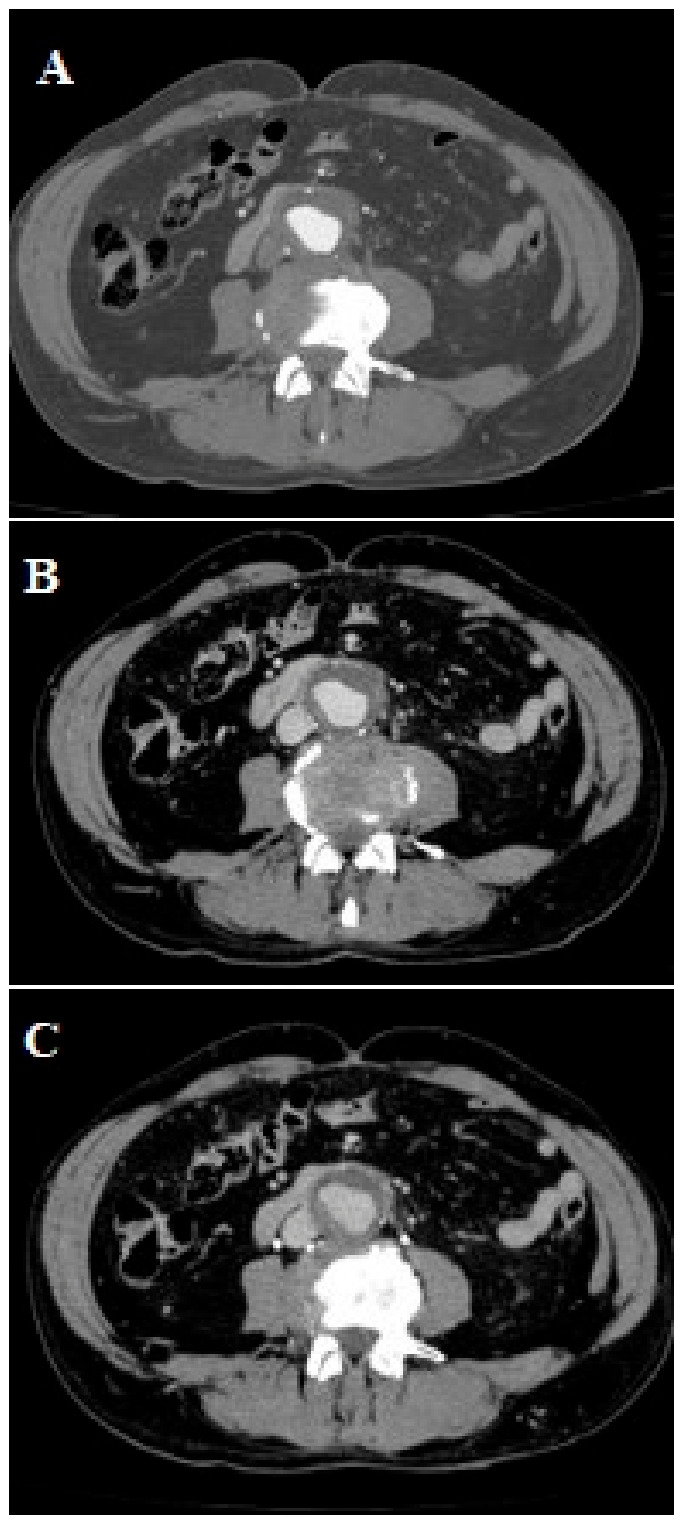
Multiphase CT angiography ((A–C): arterial-phase, axial plane at different level) demonstrates a fusiform infrarenal abdominal aortic aneurysm with irregular mural thrombus and focal wall bulging. These features reflect heterogeneous wall remodeling and potential instability, highlighting the value of multiphase CTA in aneurysm characterization and risk assessment.
